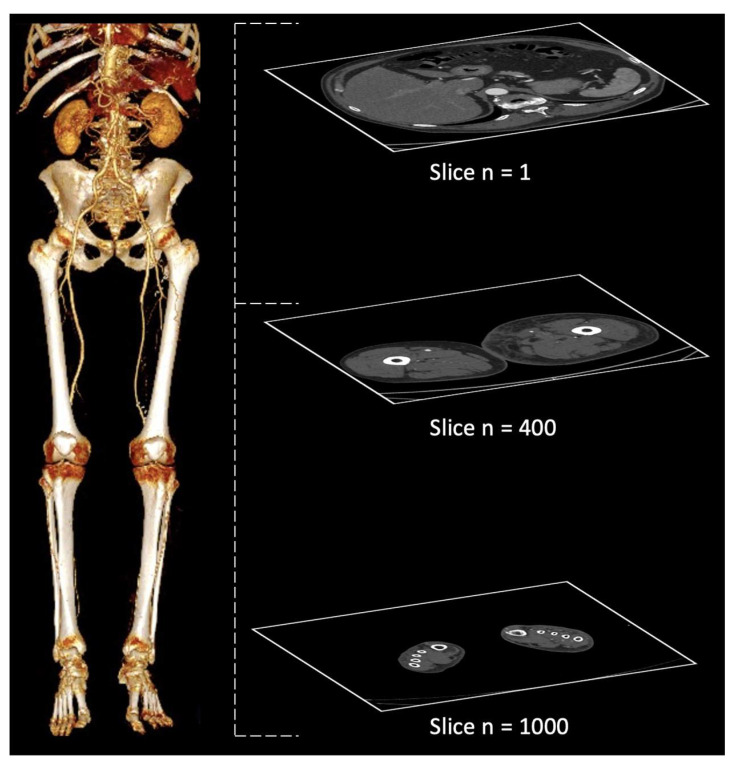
Example of a full 3D CTA scan of the lower limbs from slice 1 (proximal) to slice 1000 (distal): on the left, reconstructed volume-rendered images displaying the arterial tree; on the right, single slices at different levels.
